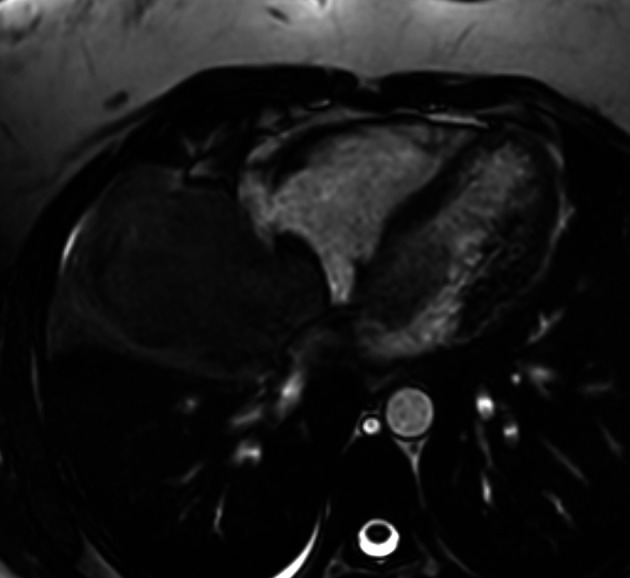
Cardiac MRI (axial cine-MRI sequence): prolapse of the mass of LA via the mitral valve.
